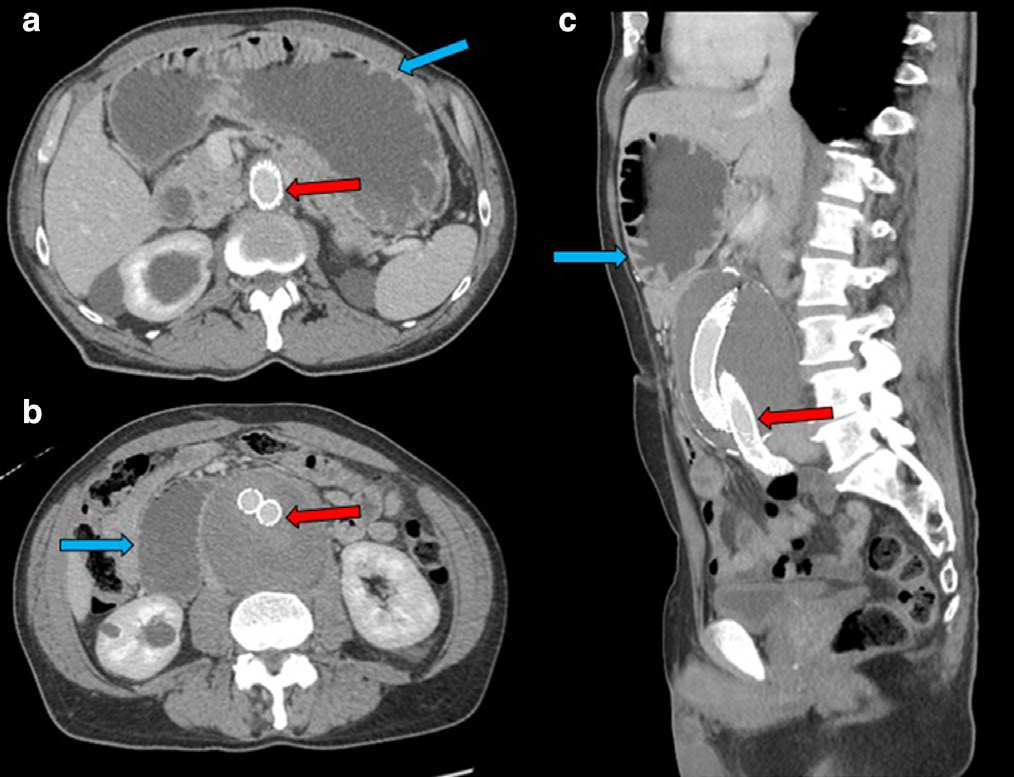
CT abdomen and pelvis postcontrast venous phase with axial views in figure 2A and 2B, and sagittal view in figure 2C, showed status postabdominal aortic aneurysm endovascular stent graft repair (Fig 1A, 1B, 1C) (red arrows) with persistent fluid filled dilatation of proximal duodenum and stomach (Fig 1A, 1B, 1C) (blue arrows).
